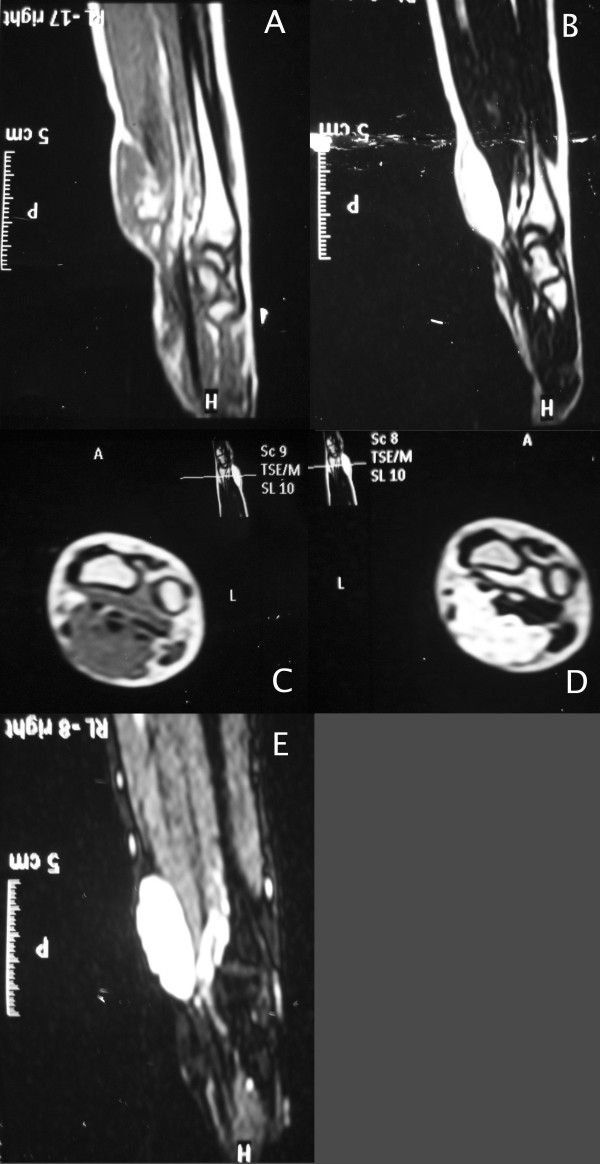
Magnetic resonance image of intraneural hemangioma. (A) Sagittal T1 (B) T2 (C) axial T1 and (D) T2 (E) fat suppression images demonstrating an 3 × 2 × 1.5 cm lesion in the volar aspect of the right wrist.
